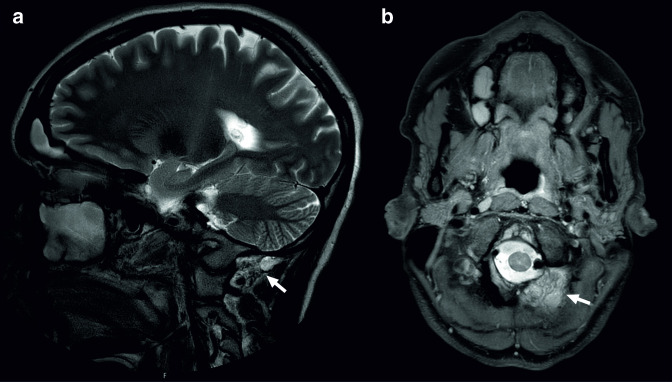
A 32-year-old female presented with frequent headaches, for which MRI of the brain was ordered to exclude any underlying pathology. MRI showed no intracranial pathology, but only signs of sinusitis. MRI also showed a soft-tissue lesion below the left occipital bone, adjacent to the left vertebral artery, as shown on *T*
